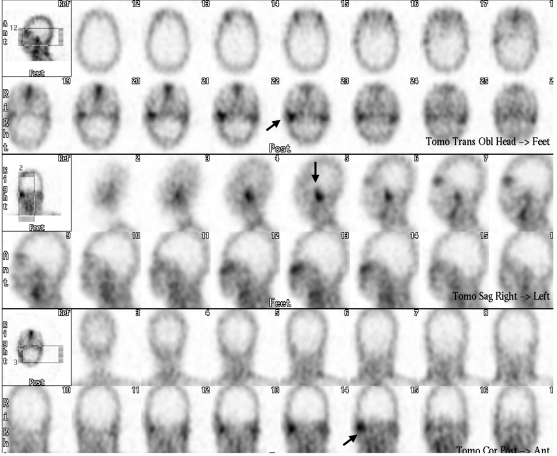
SPECT ^67^Ga scintigraphy images showing significantly increased uptake in the right petromastoid region. (black arrow).
